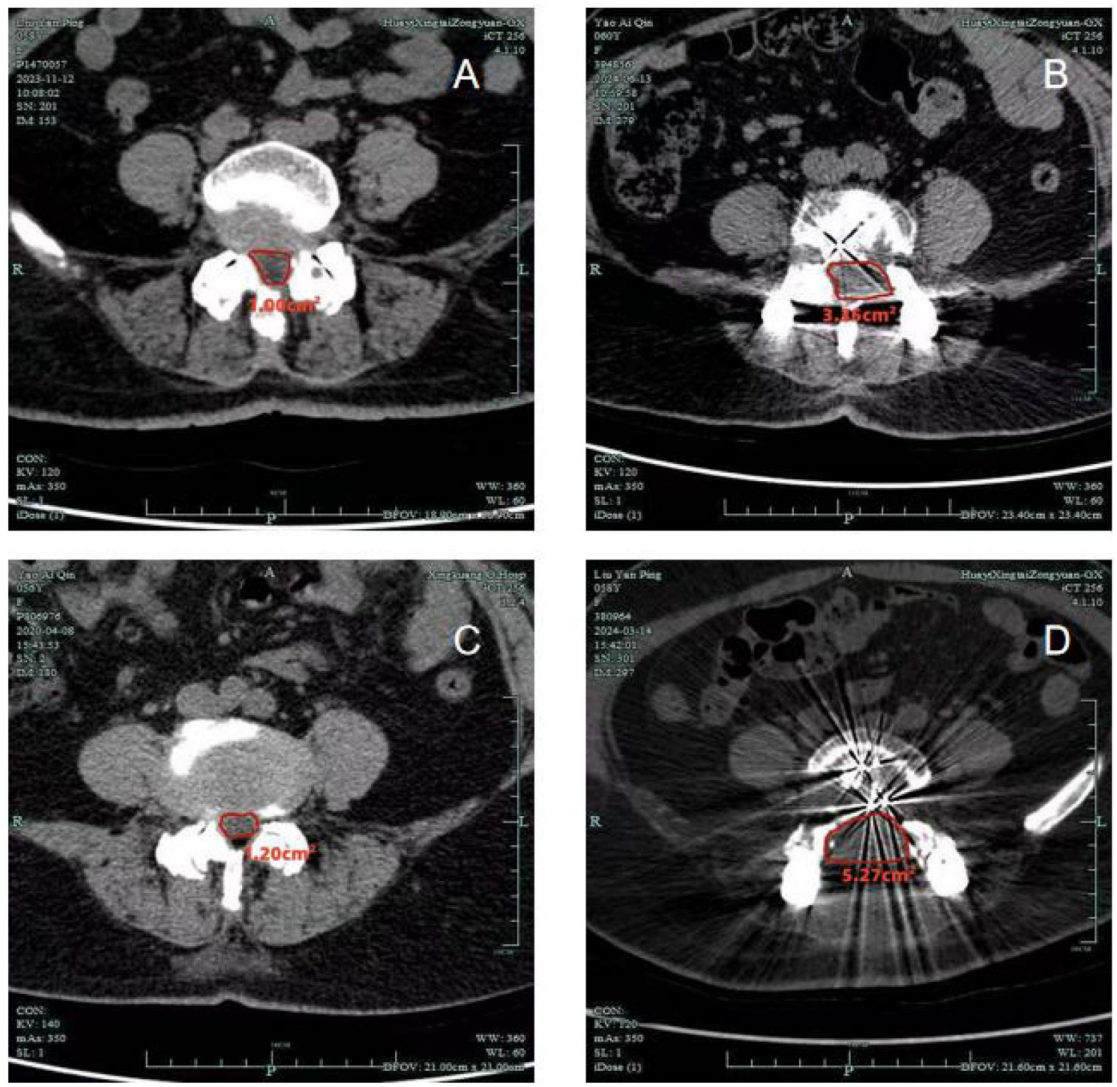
Computed tomographic scans showing the dural sac cross-sectional area, measured using an automated digital tool in the PACS system. [(A) Unilateral laminotomy, Preoperative; (B) unilateral laminotomy, Postoperative; (C) conventional laminectomy, Preoperative; (D) conventional laminectomy, Postoperative].
